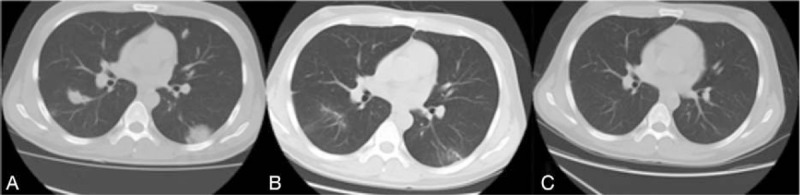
Chest computed tomography (CT) scan at baseline (A), 2 weeks (B), and 2 months after treatment (C), indicating the disappearance of pulmonary consolidation.
